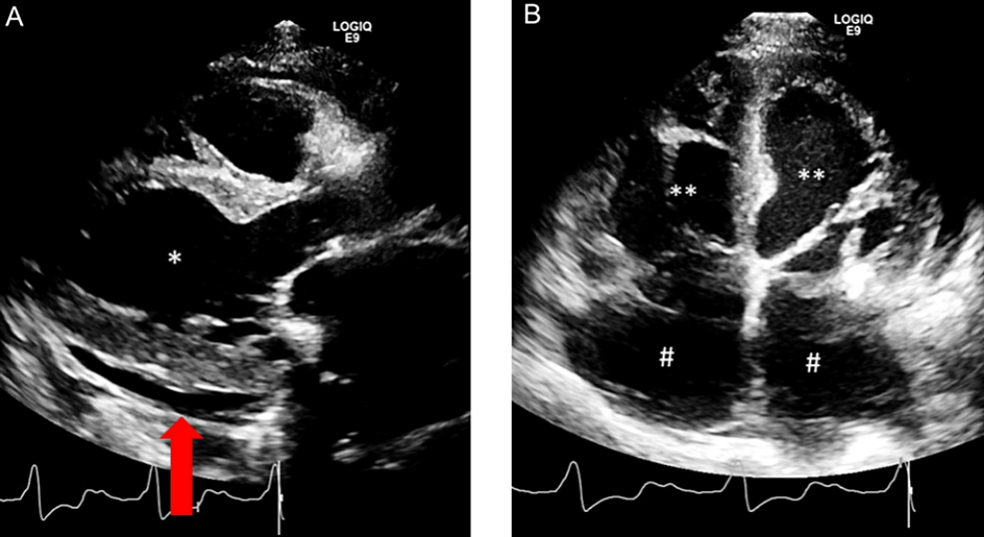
A: Parasternal long axis showing left ventricular concentric (*) and eccentric hypertrophy (*) and mild pericardial effusion (indicated by the red arrow). B: Four-chamber views showing left and right ventricular concentric () and eccentric hypertrophy (), bilateral atrial enlargements (#), and mild pericardial effusion.
